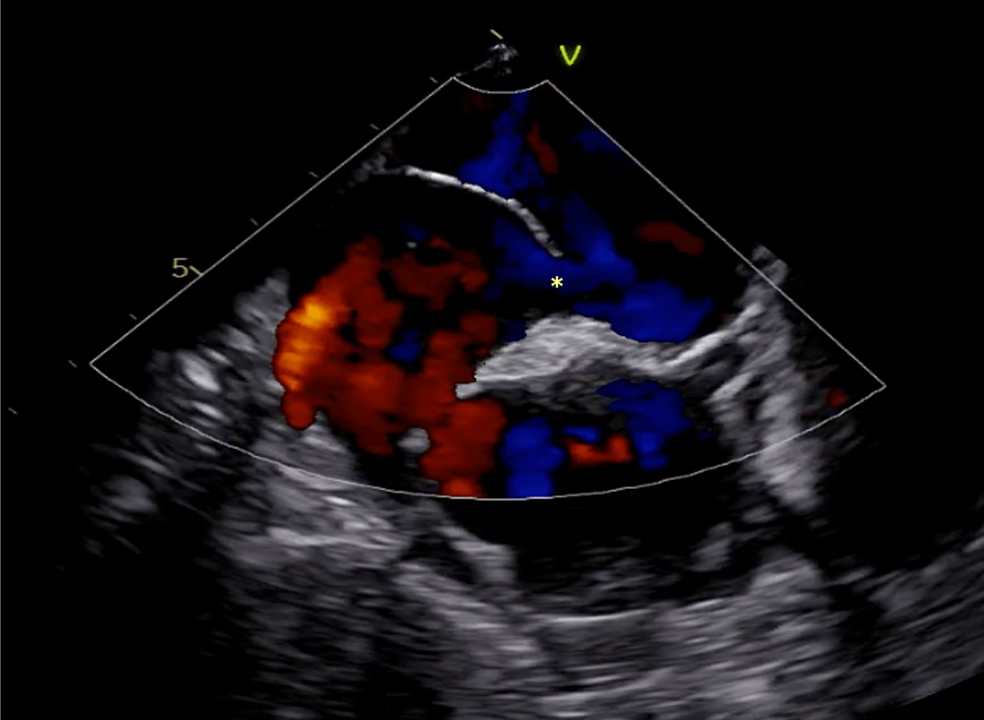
Transesophageal echocardiogram. A large patent foramen ovale (*) with a bidirectional shunt was noted in the transesophageal echocardiogram.
